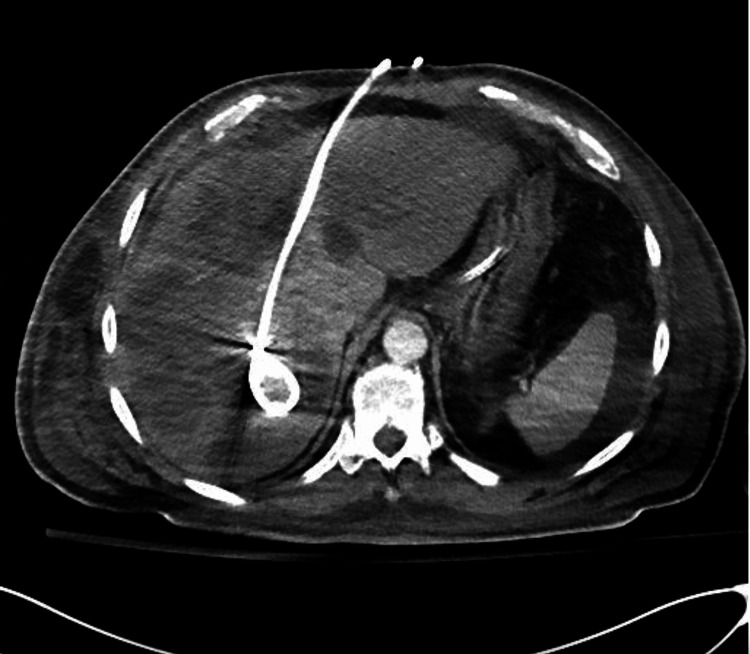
CT scan of the abdomen percutaneous drainage was performed with a catheter in place CT, computed tomography.
